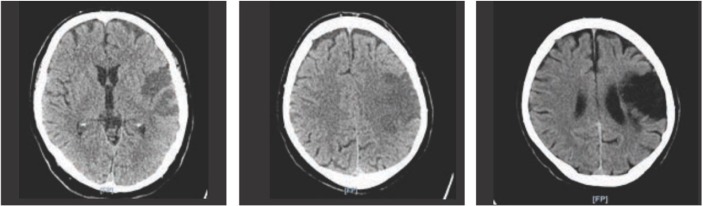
CT labeled A and B showing early ischaemic changes 24 hours after thrombectomy. CT labeled C was done at one-year follow-up.
